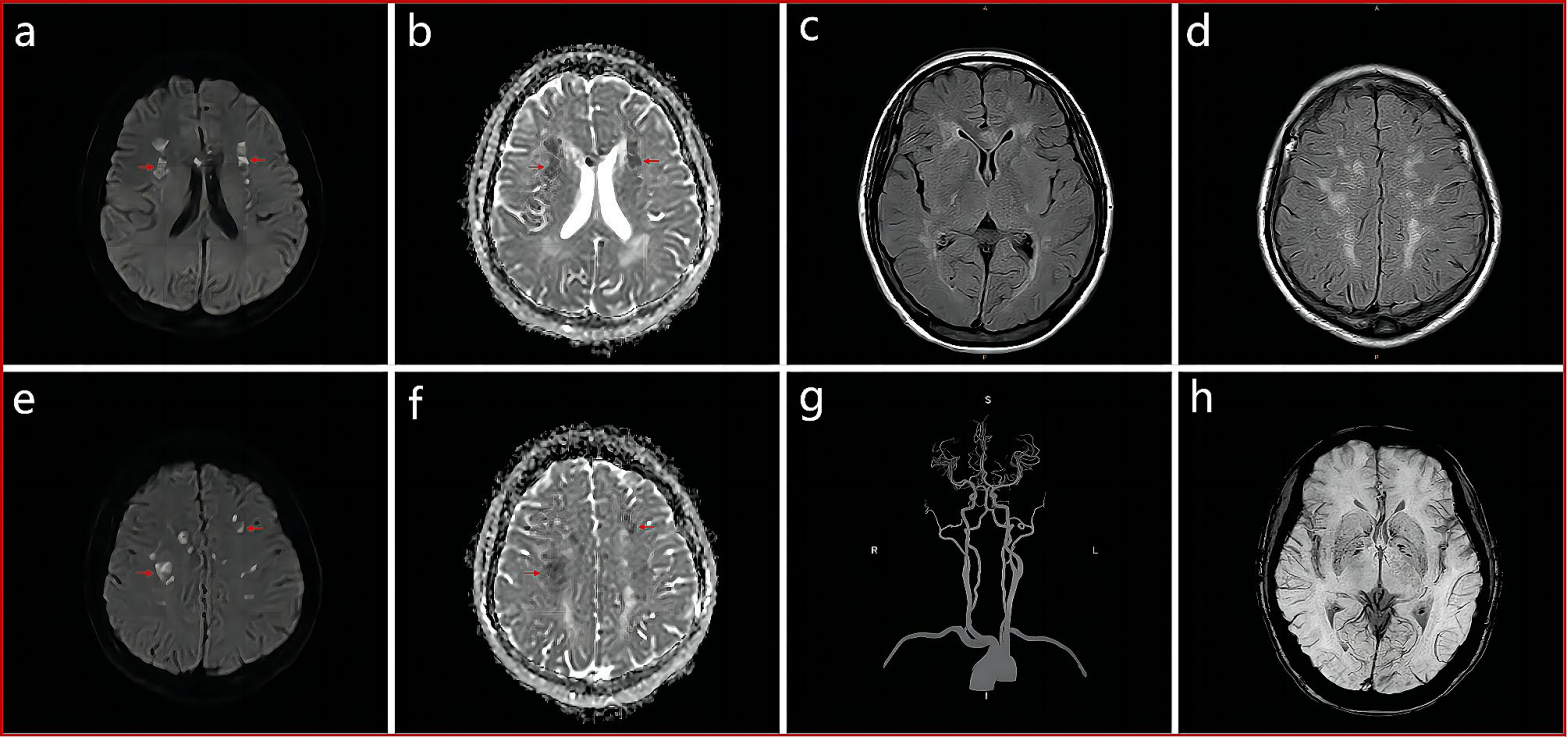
Acute bilateral multiple subcortical infarcts in patient (a, b, e, f). White matter demyelination (c, d). CT angiography (g). SWI (h)
